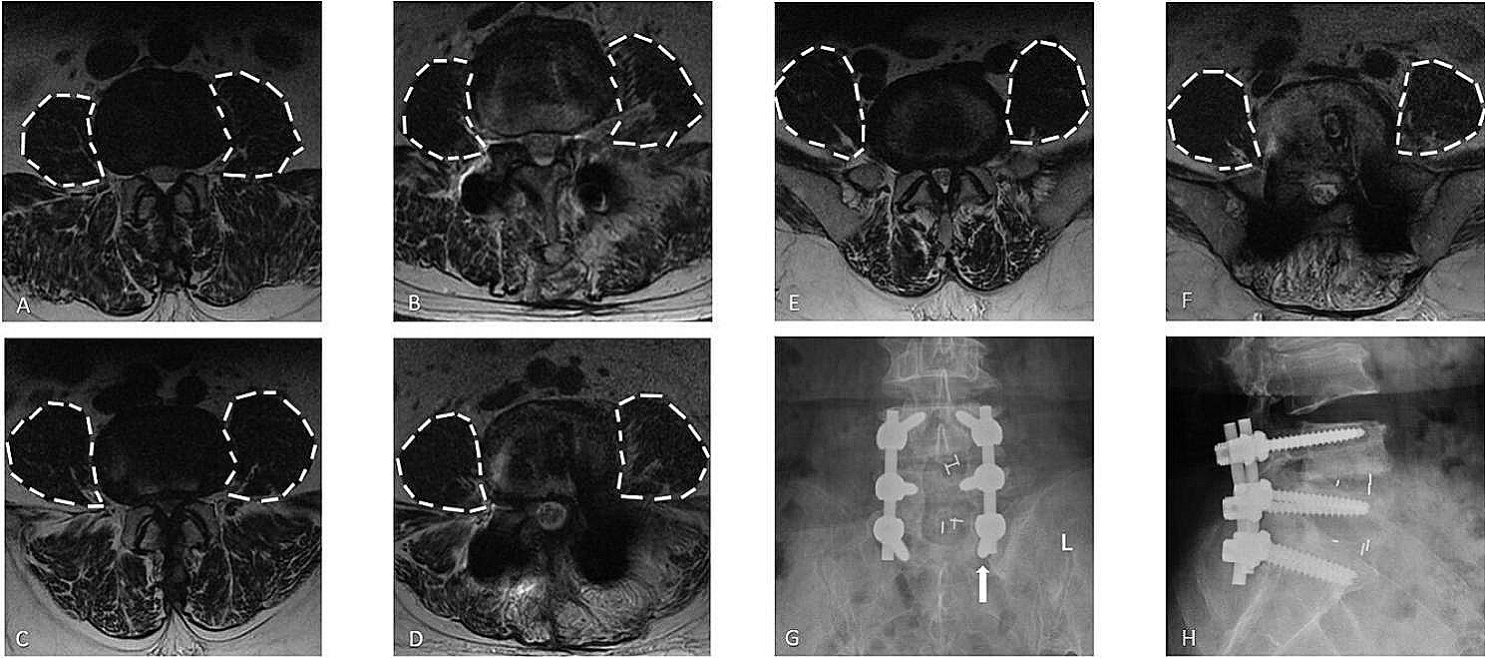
Preoperative and postoperative radiographs of a 59-year-old woman presenting with first sacral vertebra (S1) screw loosening at the 30-month follow-up (G, H). Preoperative functional cross-sectional areas (fCSAs) of the psoas major muscle (PS) at L3–4 (A), L4–5 (C), and L5–S1 (E) are shown. Postoperative fCSAs of the PS at L3–4 (B), L4–5 (D), and L5–S1 (F) are shown. Moreover, the ratio of postoperative and preoperative rfCSA at L3-S1 was calculated as 0.89, which was lower than the proposed 1.075 cutoff value
